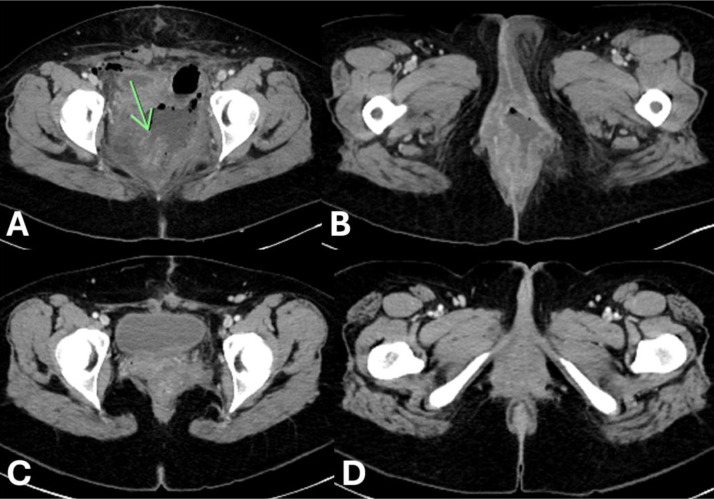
Contrast-enhanced CT of the abdomen and pelvis performed after resection of the AAM showed a pelvic fluid collection with air secondary to iatrogenic rectal injury (A). The procedure was also complicated by a rectovaginal fistula (green arrow) that allowed feculent material to enter the vaginal canal (B). The most recent follow-up CT scan (C, D) demonstrated resolution of these findings 42 days after resection.
